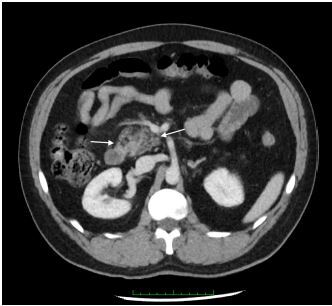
Axial computed tomography view showing inflammatory stranding of the pancreatic head (arrows).
